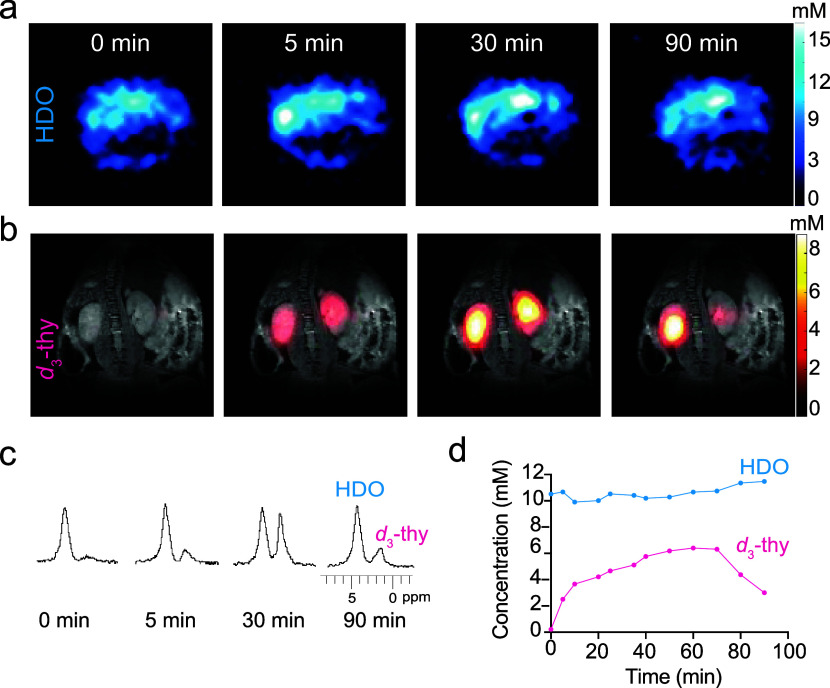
In vivo*^2^H-MRI mapping and quantification
